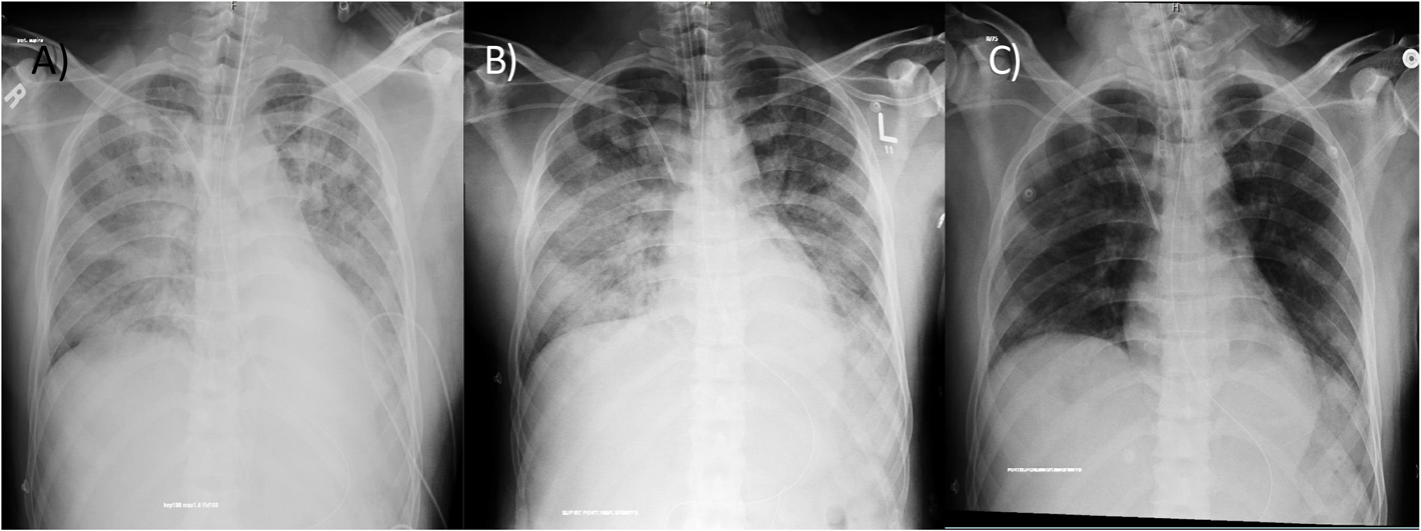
Case 65: a 38-year-old male, with mild obesity, no other comorbidities, score 7 under vasopressor treatment. Chest X-ray: a day of inclusion (April 16th). Extensive consolidations involving both lung fields with left retrocardiac volume loss. Blunting of the left CP angle. Ill-defined opacities seen in bilateral lower zones. b Day 4 after treatment (April 20th). Improved appearance of patchy airspace opacity in the whole left lung with almost resolved bilateral pleural effusion and underlying atelectasis; however, consolidation is again noted in the left retrocardiac area with mild worsening of airspace opacity in the right mid and lower zones. c Day 9 after treatment (April 25th). Regression of patchy airspace opacification mid-right and left lung when compared to the previous study. Residual patchy bilateral airspace opacification particularly in the left retrocardiac region
